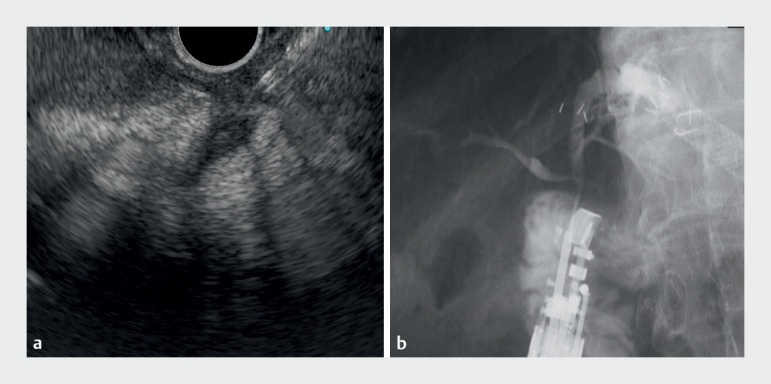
Endoscopic ultrasound and fluoroscopic images showing:
